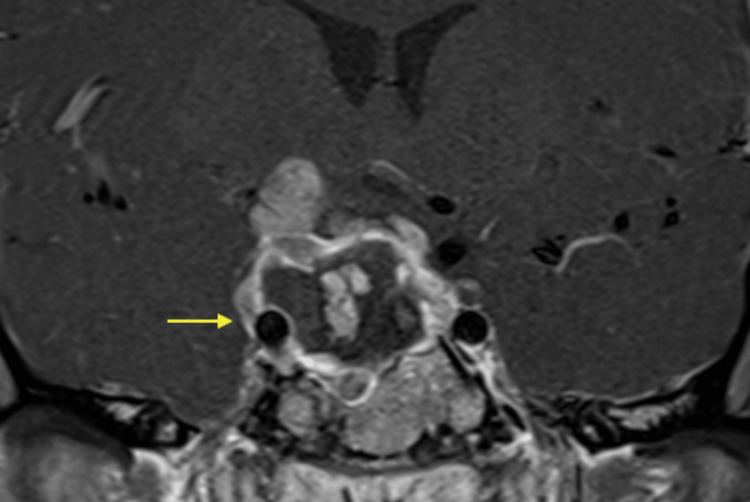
Postoperative coronal MRI of pituitary mass T1 post-contrast showing subtotal resection of previously described sellar/suprasellar mass. Residual enhancing tumor along the right aspect of the sella and right cavernous sinus (yellow arrow) extending to the suprasellar region. There is encasement of the right internal carotid artery.
